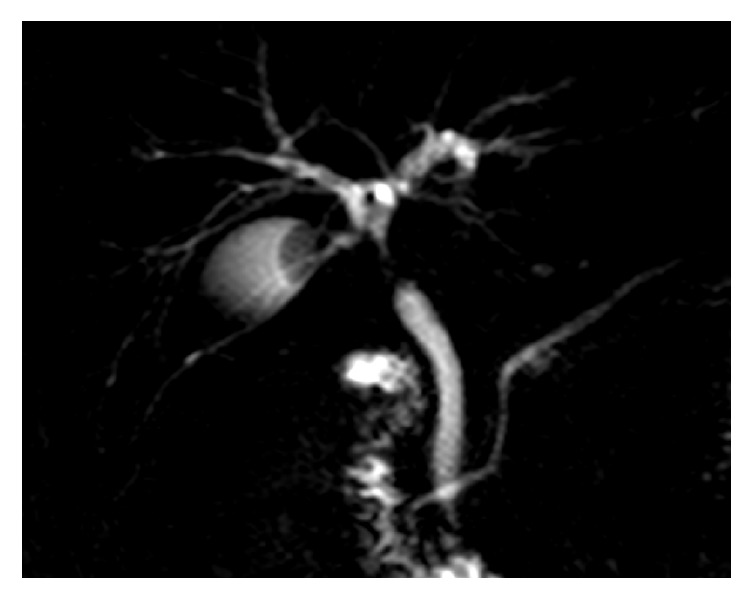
MRI cholangiography in a patient with Mirizzi type I mimicking a biliary cancer.
